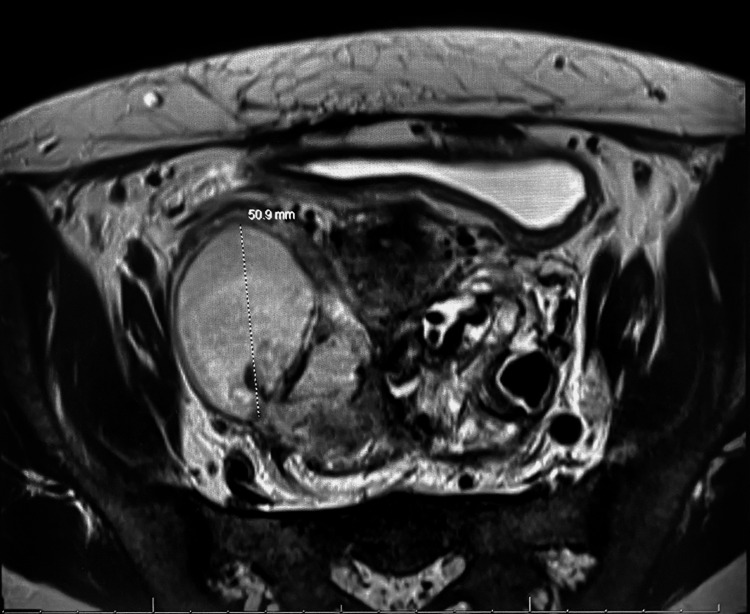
T2-weighted axial MRI of the pelvis demonstrating a large complex adnexal fluid collection measuring approximately 50.9 mm, notable inflammatory changes, and adjacent bowel loops suggest secondary involvement.
